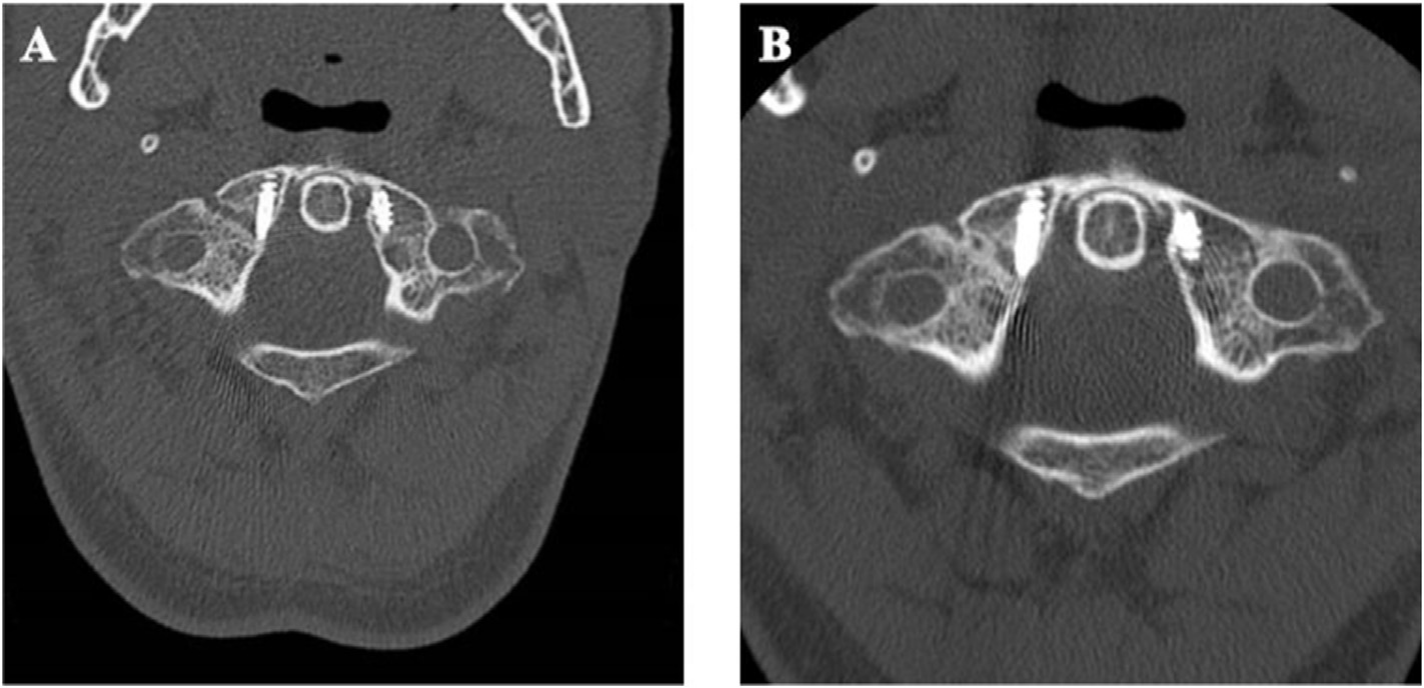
(A–B) CT scan follow up at 3 and 9 months respectively with good reduction and bone edge fusion.
